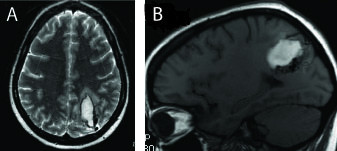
Magnetic resonance imaging of the left parieto-occipital arteriovenous malformation. T2-weighted magnetic resonance imaging scans of the malformation and associated hematoma from the outside hospital showing (A) axial and (B) sagittal views.
